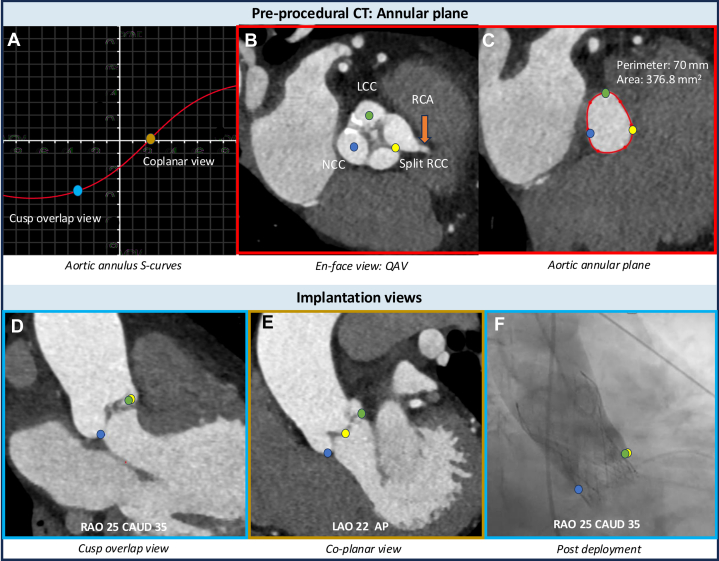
CT-Guided Procedural Planning and Fluoroscopic Angles in Quadricuspid Aortic Valve (A) CT-derived aortic annulus S-curve (red), with cusp-overlap view (blue dot) and co-planar view (brown dot). (B) En face view of the aortic valve showing left coronary cusp (LCC, green), non-coronary cusp (NCC, blue), and right coronary cusp (RCC, yellow); the RCC was functionally treated as a single cusp despite being split, to facilitate obtaining the annular plane. (C) Nadir points of the LCC, NCC, and split RCC used to obtain the annular plane. (D) Cusp-overlap view (RAO 25°, caudal 35°) isolating the NCC and overlapping LCC and RCC for deployment depth guidance. (E) Co-planar view (LAO 22°, AP) aligning all 3 cusps in a single plane. (F) Final valve deployment performed in cusp-overlap view (RAO 25°, caudal 35°) using the NCC as depth reference. CAUD = caudal; LAO = Left anterior oblique; LCC = left coronary cups; NCC = non-coronary cusp; RAO = right anterior oblique; RCC = right coronary cups.
